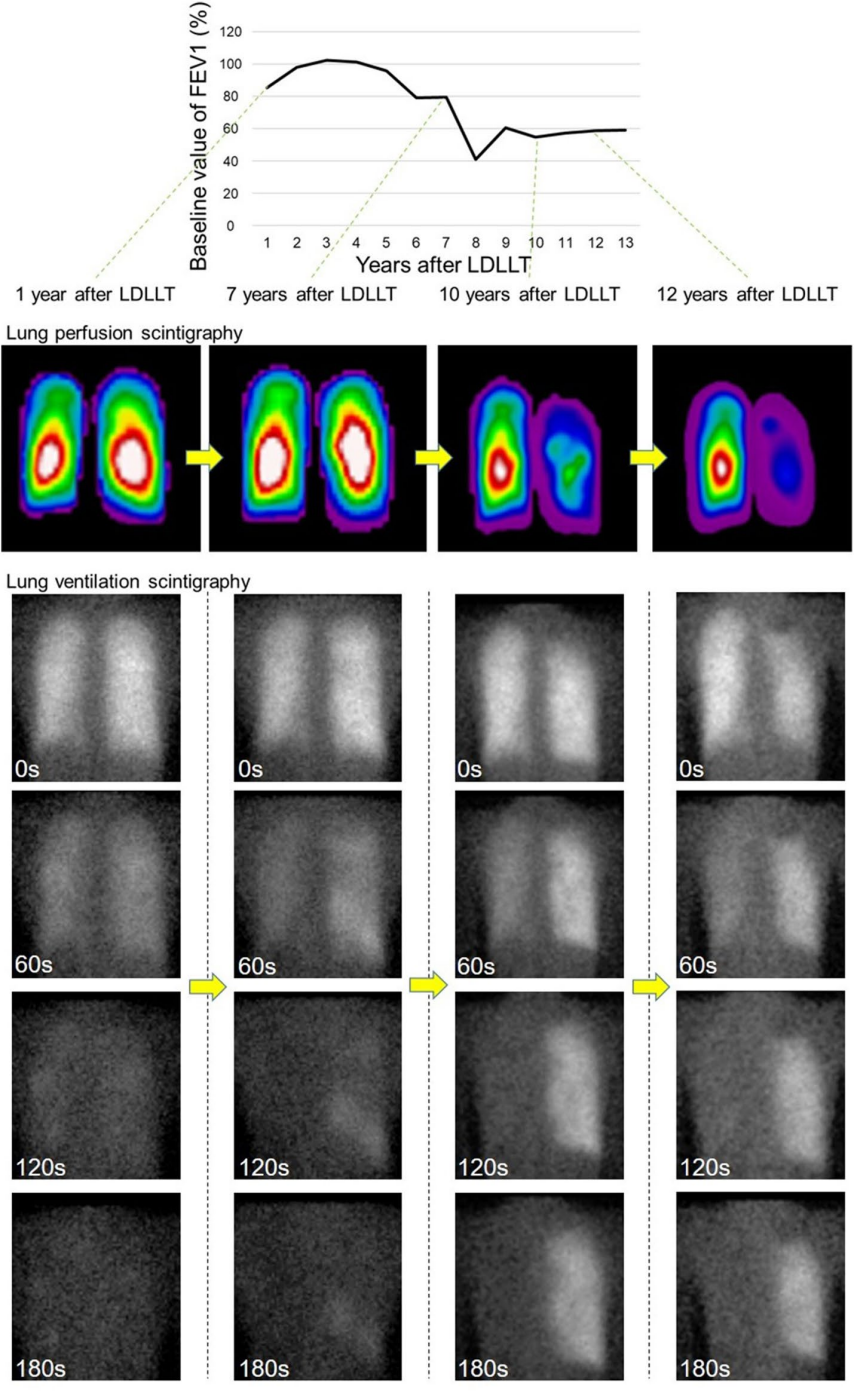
Representative course of unilateral chronic lung allograft dysfunction (CLAD) after bilateral living-donor lobar lung transplantation (LDLLT). Lung perfusion scintigraphy demonstrated a perfusion shift to the contralateral unaffected lung in the patient with unilateral CLAD after bilateral LDLLT, which was also associated with a decline in the forced expiratory volume in 1 s (FEV1) associated with CLAD. The lung ventilation scintigraphy showed that the washout of radionuclide tracer became slower, indicating air trapping in the lung affected with unilateral CLAD.
